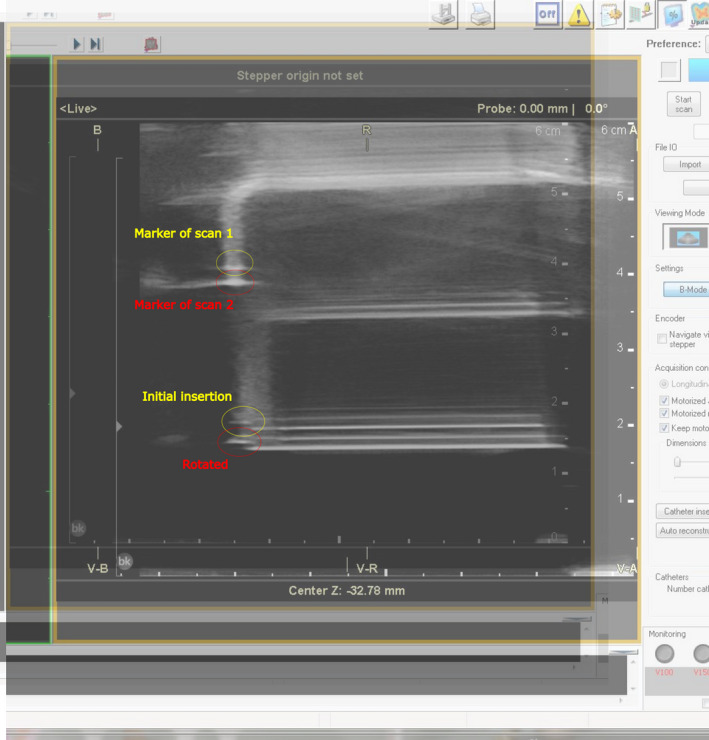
Sagittal ultrasound (US) images of the in‐house machined US maker and an interstitial needle. Two images are registered in depth to the center of the US marker but displaced vertically to showcase the differences in the needle's measured position from the US due to the needle being rotated
